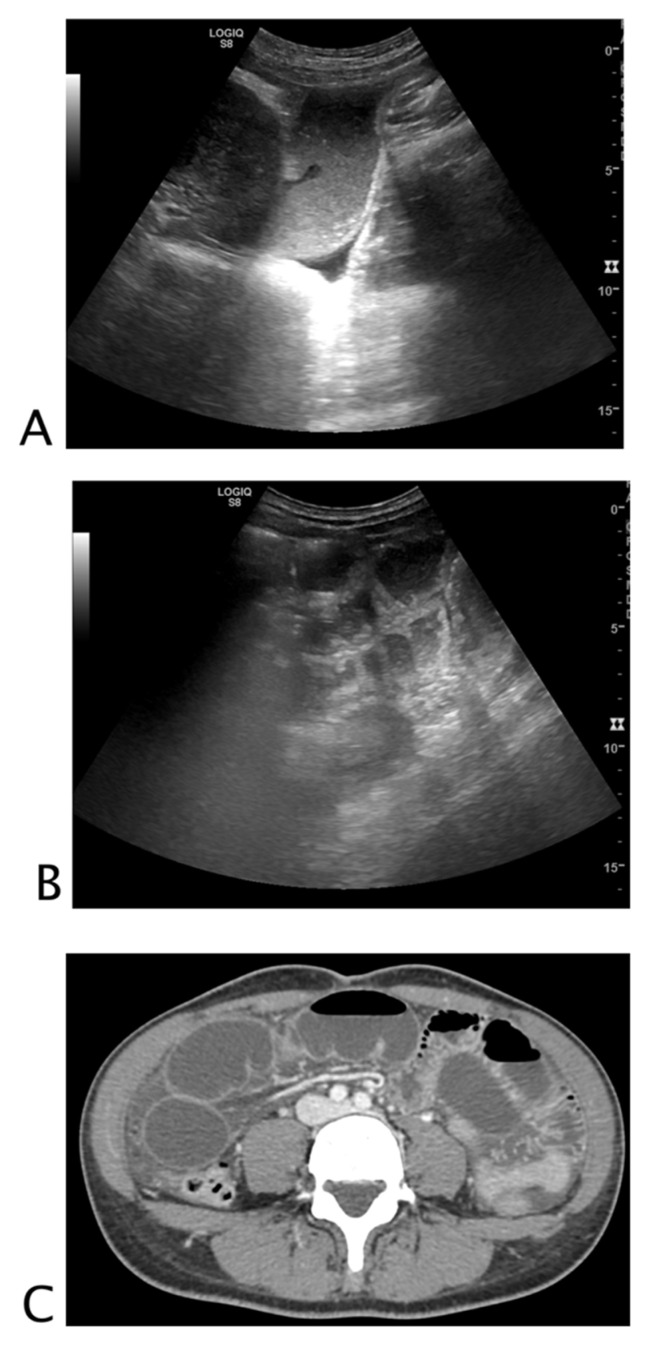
Black is too much, and it is where it should not be. A 68-year-old woman with abdominal distension, pain, and vomit. At ultrasound, (A) hypokinetic dilated small bowel loops were visualized on the right flank with thin parietal walls and poor evidence of valvulae, and free fluid was detected between bowel loops. On the left flank, (B) fluid between undilated bowel loops with regular representation of valvulae were detected. A suspected diagnosis of decompensated mechanical small bowel obstruction with fulcrum on the right flank was formulated. The patient underwent abdomino–pelvic CT; (C) pelvis that confirmed mechanical obstruction determined by adhesions on the right flank. Final diagnosis: decompensated small bowel obstruction.
